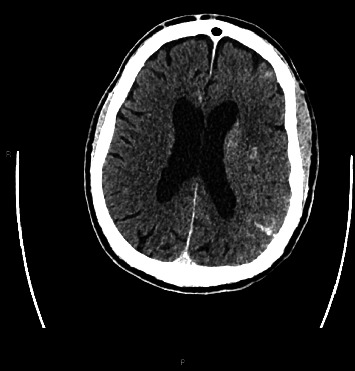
Postcontrast CT demonstrating extensive hyperdense cortical oedema with contrast enhancement and loss of grey–white matter differentiation consistent with CIE.
